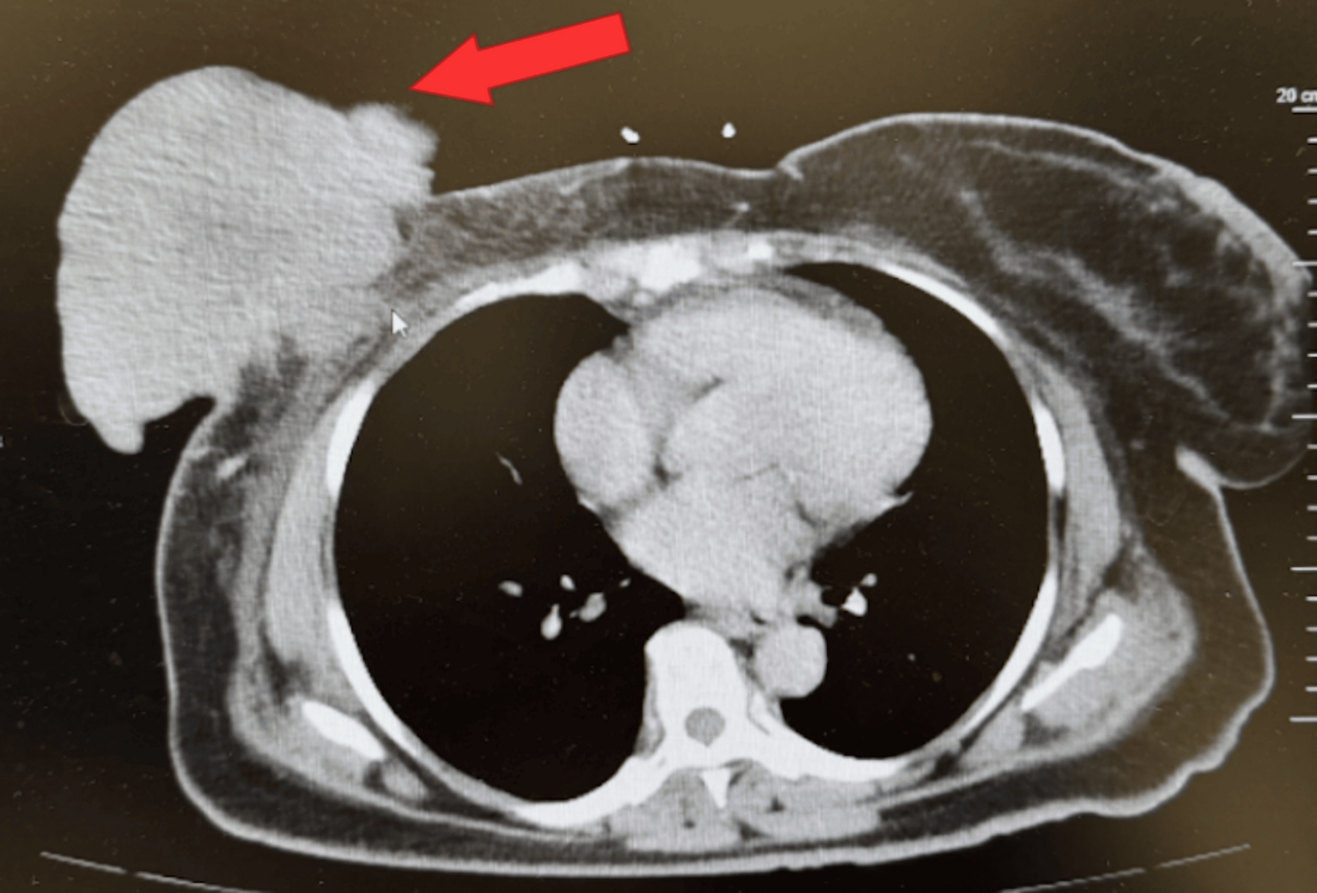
Contrast enhanced CT scan of the chest, abdomen, and pelvis demonstrating a large exophytic right breast mass (red arrow).
